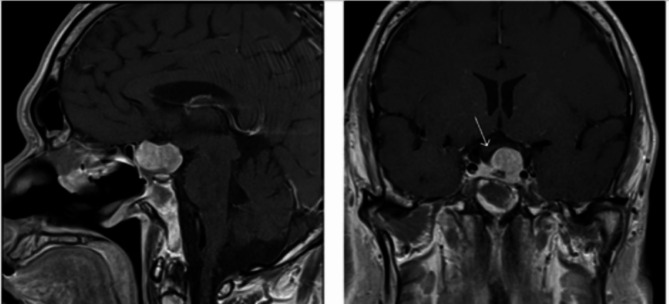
Follow-up Magnetic Resonance Imaging (MRI) of pituitary gland 1-year post operatively showing regression in lesion size. The optic chiasm is decompressed (arrow)
